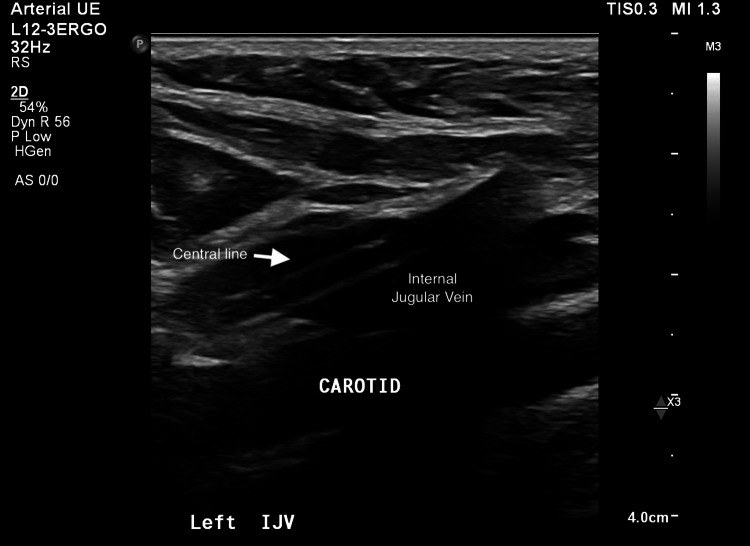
Ultrasound confirmation of central line positioning within the internal jugular vein with the carotid patent IJV: internal jugular vein (long axis view)
